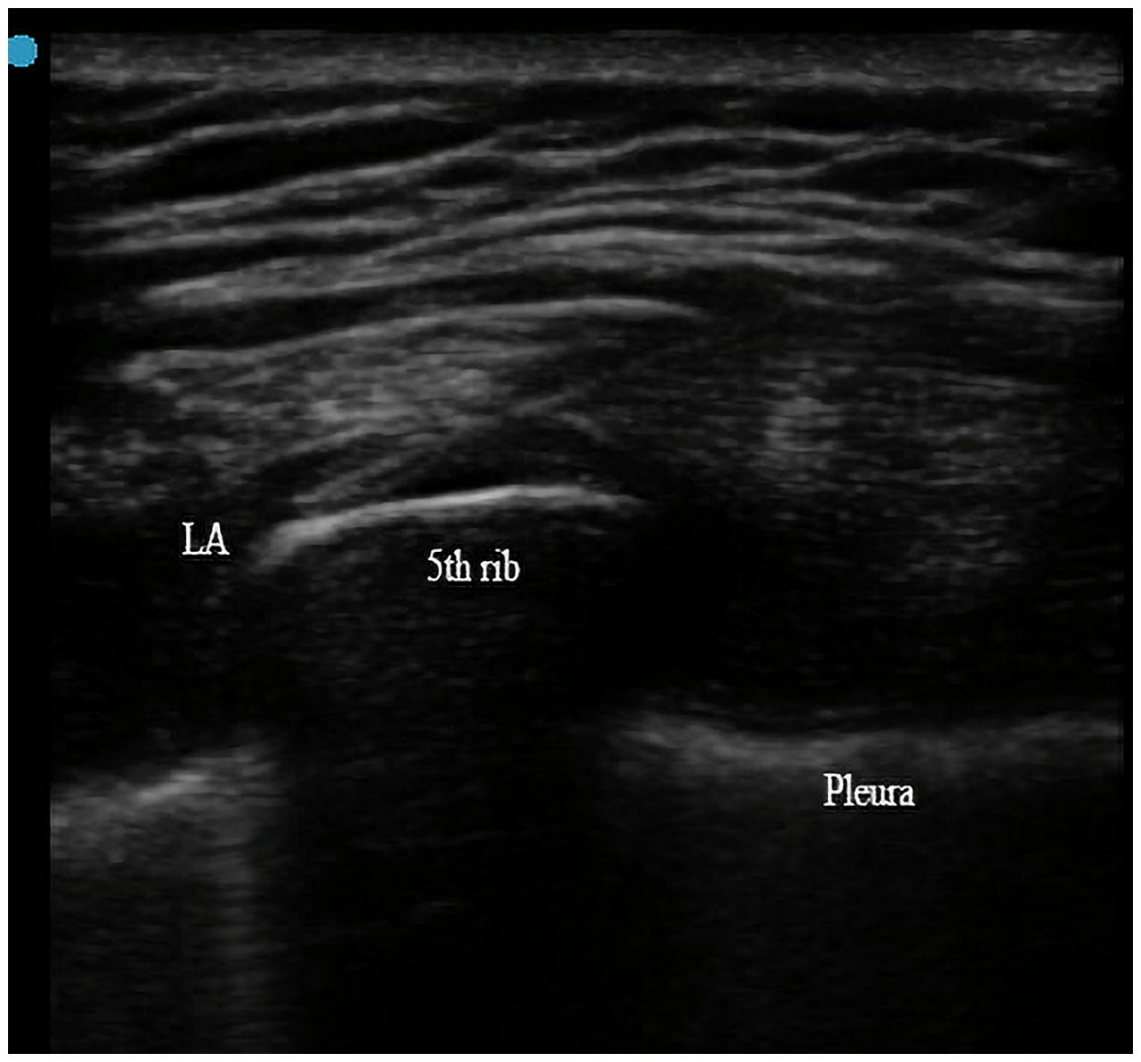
Local analgesic (LA) was injected into the plane below the serratus anterior muscle and above the fifth rib with the ultrasound guidance.
